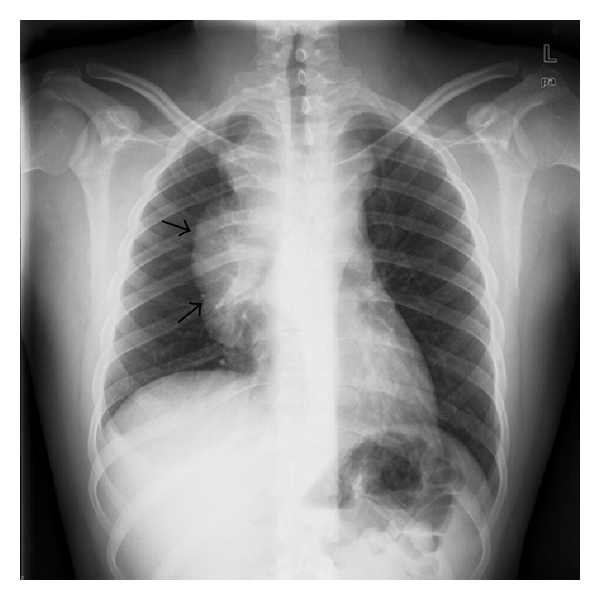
Chest radiograph at initial presentation showing large right hilar mass with right paratracheal soft tissue mass (see arrows).
